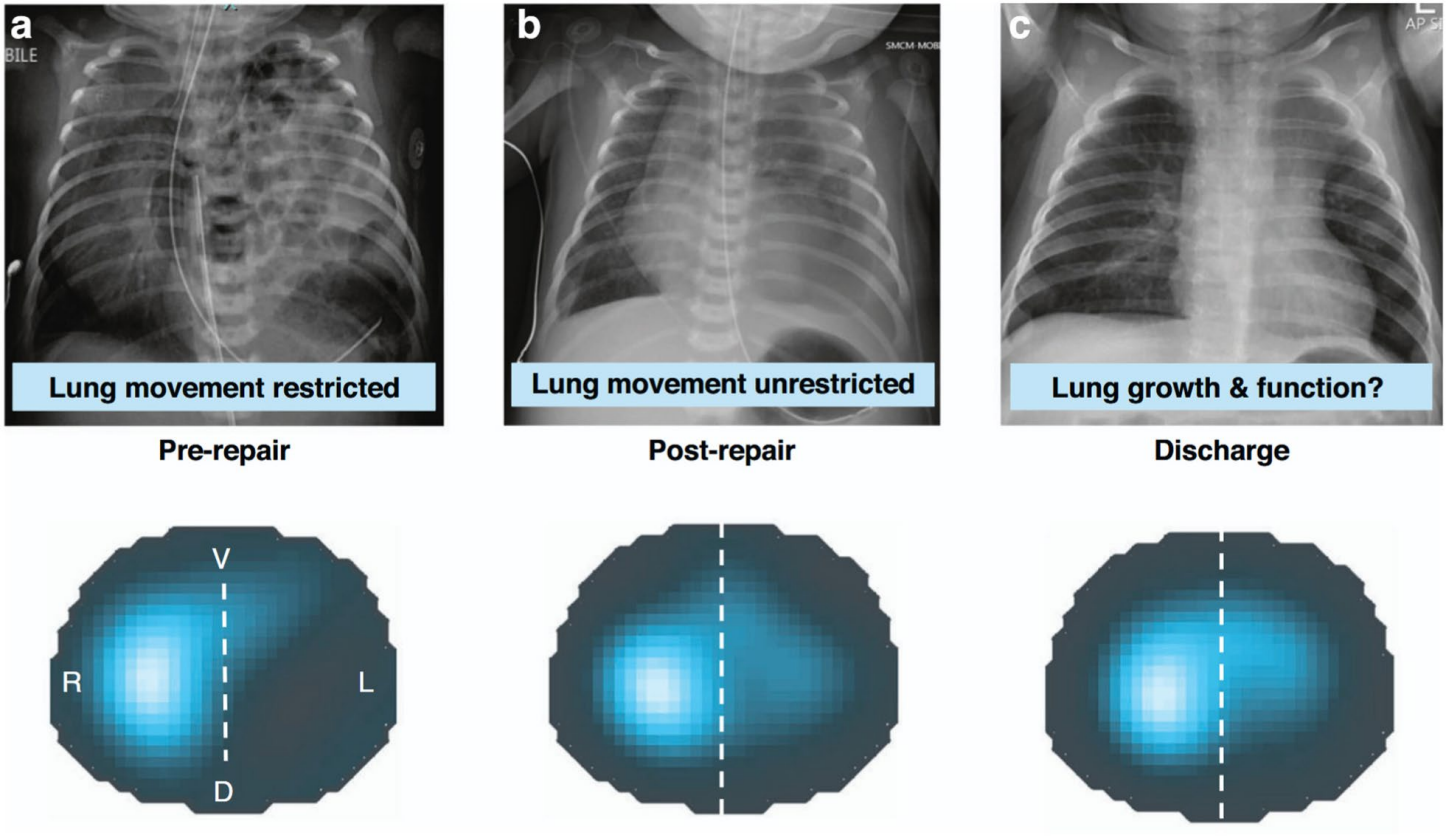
Chest radiography and functional EIT images acquired before and after the surgical repair of congenital diaphragmatic hernia. First lung imaging was performed before surgery (a). At this timepoint the left (ipsilateral) lung was hypoplastic and lung movement in both lungs (but especially the left) was restricted by hernia contents in thorax. Second lung imaging (b) was performed as soon as possible (defined by clinical stability) after the hernia repair. At this point the left lung was likely to be as equally hypoplastic as in pre-repair state, but movement was not restricted by herniated contents in thorax. Pre-discharge imaging (c) was undertaken at time clinical team commenced discharge planning. Magnitude of tidal ventilation in each lung region is expressed using a colour scale from dark blue (minimum) to light blue/white (maximum). R: right, L: left, V: ventral, D: dorsal (dotted line demarcates ipsilateral [left] and contralateral [right] hemithorax). The figure is reproduced under a Creative Commons Attribution 4.0 International License from [62]
